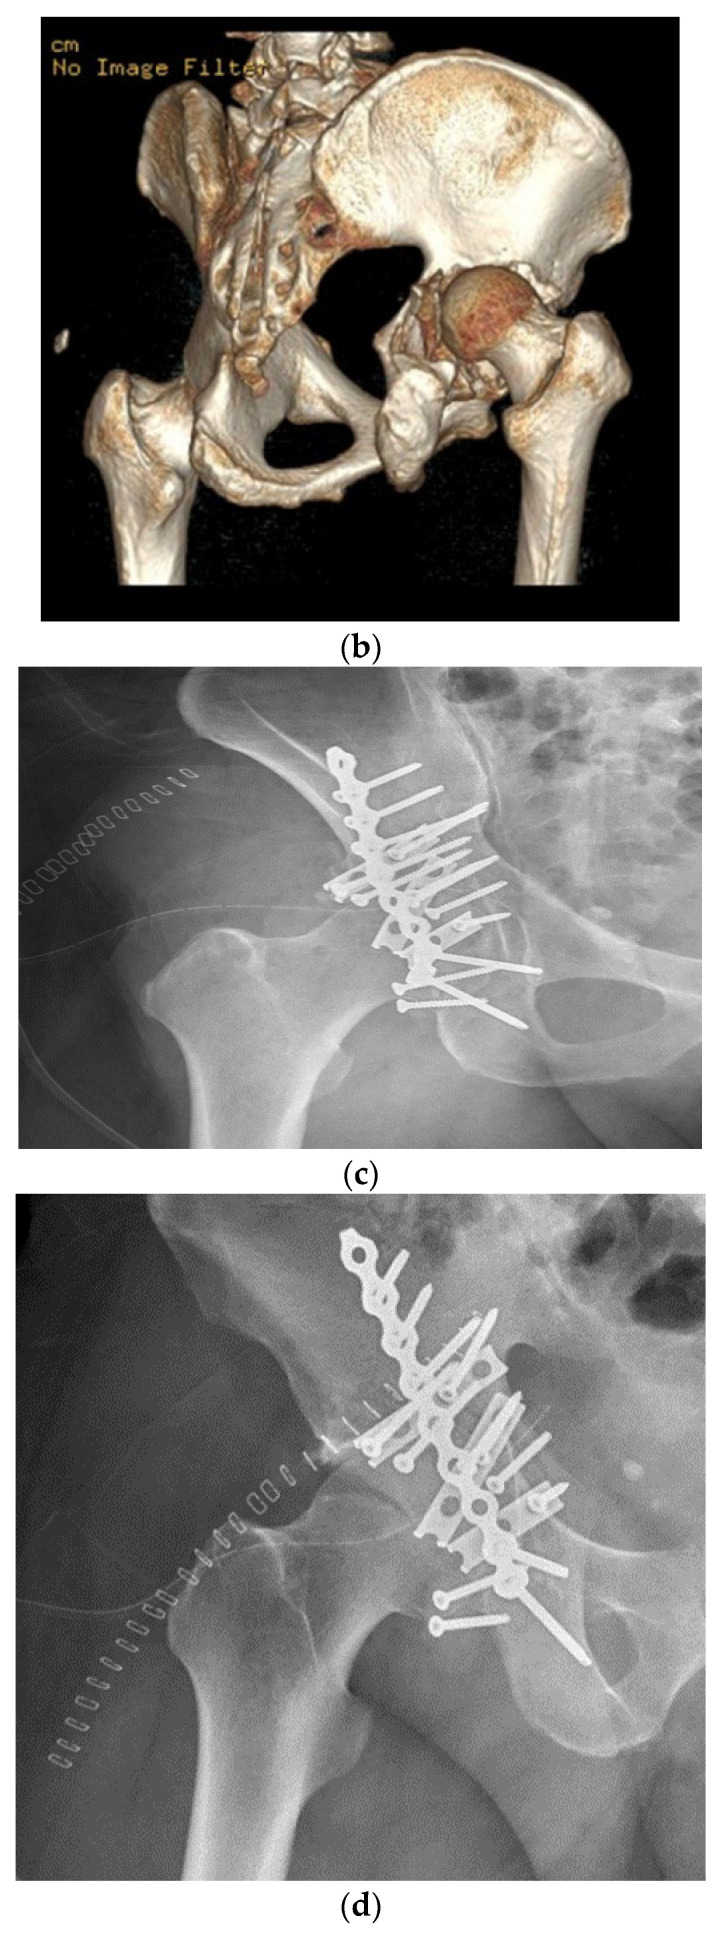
A 55-year-old male patient with injury to the right hip in a traffic accident. Preoperative radiography and CT scan reveal comminuted posterior right acetabular wall fracture associated with dome comminution (a,b). Open reduction and internal fixation with additional three spring plates have been performed to stabilize the comminuted fragments. Postoperative radiography shows good reduction of the acetabular joint surface (c,d).
